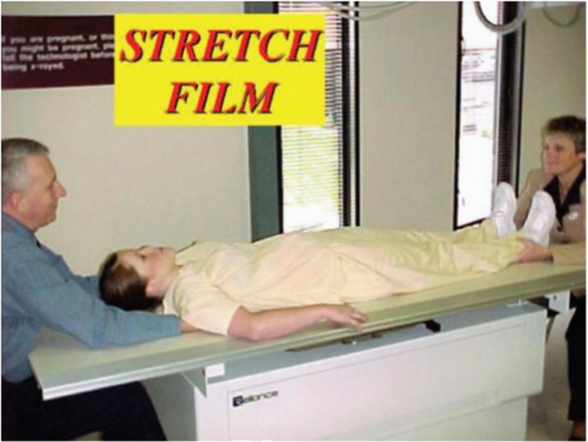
Performing a “Stretch film”. The patient is laid supine on the radiograph table and a 36-inch film is placed under the patient. Two trained clinical persons then “stretch” the patient gently while the patient is instructed to relax and enjoy the experience.
